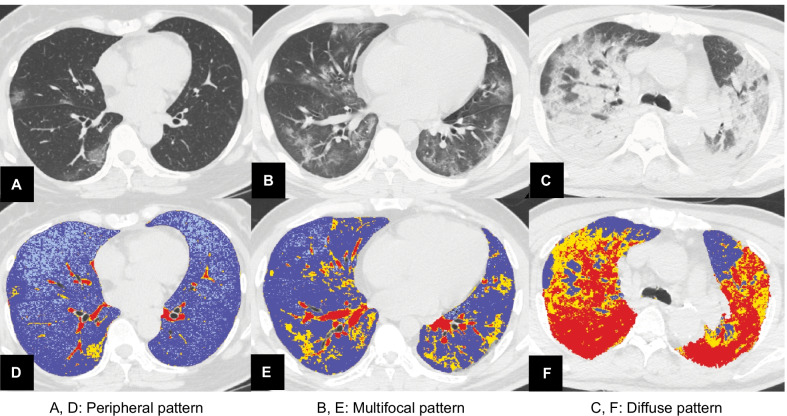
Computed tomography (CT) images for the three patterns of COVID-19 pneumonia. The pulmonary opacities are classified into peripheral, multifocal, and diffuse patterns. a In the peripheral pattern, parenchymal opacification appears in the inner peripheral zone. b In the multifocal pattern, parenchymal opacification is apparent in the central and peripheral regions. c The diffuse pattern reveals generalized pulmonary involvement, with regional inhomogeneity. d–f Semi-automated segmentation using Thoracic VCAR software (GE Healthcare, USA). Blue areas represent normal lung parenchyma in the -501, -900 HU interval; light blue areas represent hyperinflated lung in the -901, -1000 HU; yellow areas represent poorly aerated lung in the -500, -100 HU interval; and red areas represent non-aerated lung in the 100, -100 HU interval. COVID-19, coronavirus disease 2019; HU, Hounsfield unit; VCAR, Volume Computer-Assisted Reading
